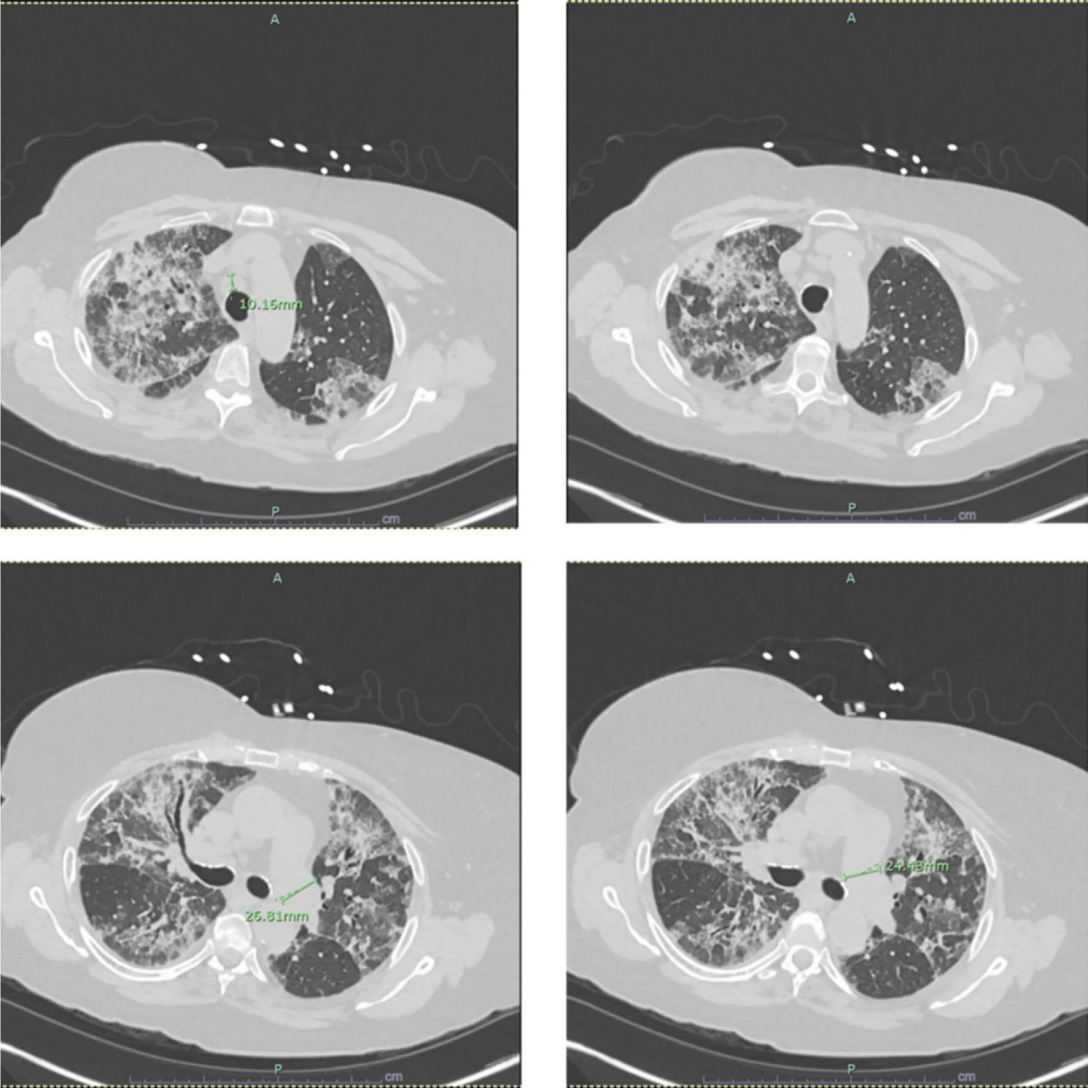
Non‐contrast Chest CT showing diffuse interstitial and alveolar opacities with upper lobe predominance.
